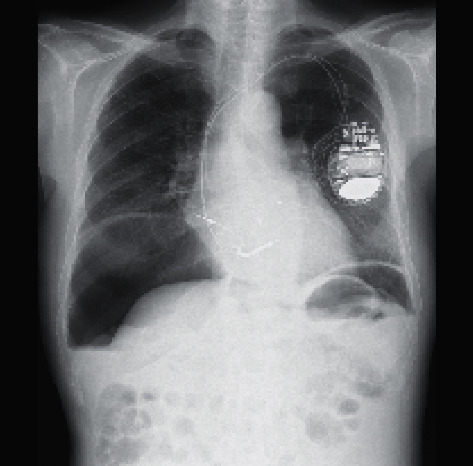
Chest X-ray upon admission. Bilateral free air in the abdomen was observed.
